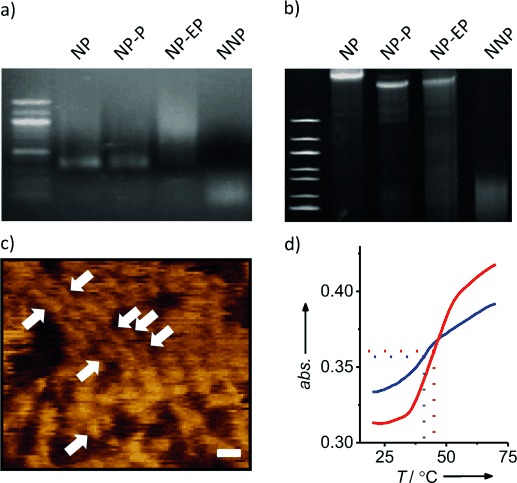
Characterization of pores NP-EP and nanostructures NP-P, NP, and NNP, assembled from DNA oligonucleotides. a) 1.1 % native agarose gel electrophoresis. Left: 100 bp marker (bp=base pair). b) 12 % SDS-PAGE. Left: 100 bp marker. c) AFM analysis of NP. Scale bar 20 nm. d) UV-melting profile of NP-PE with (red) and without (blue) SUVs.
